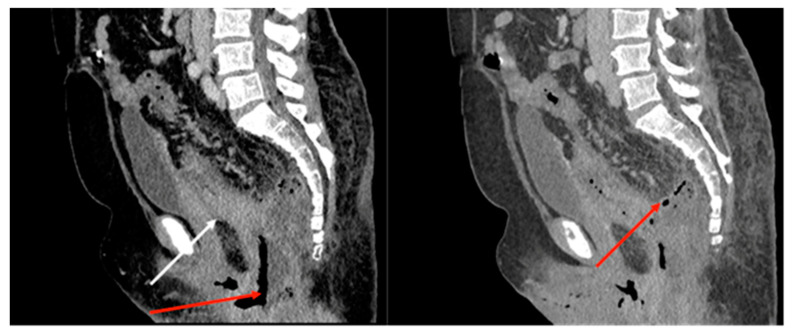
Sagittal CT of the pelvis; there is a rectal mass (white arrow) with an accompanying abscess (red arrow).
